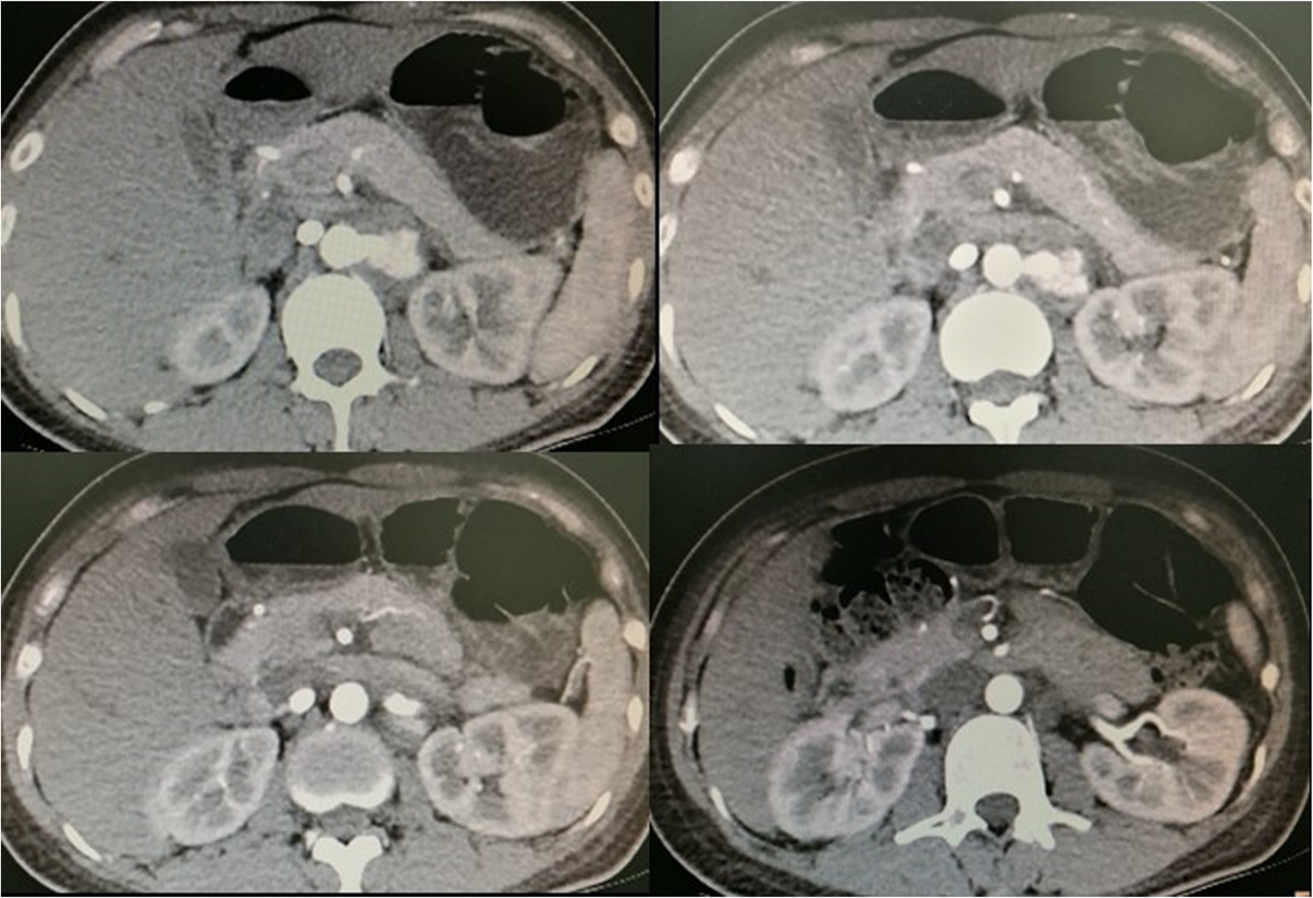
Computed tomography (CT) revealed aneurysm in the left renal artery
